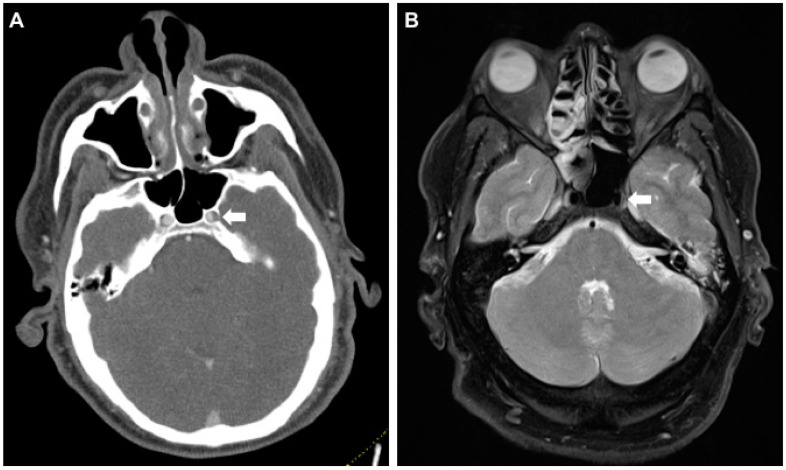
False-positive CT-A raising suspicion for an intramural hematoma of the left internal carotid artery (ICA) in its petrosal segment. (A) Axial CT-A of the skull base shows caliber asymmetry of the left ICA in the foramen carotideum (white arrow). (B) MR (proton-density fat saturated) rules out a wall hematoma. Asymmetry is presumably caused by the carotid plexus of nerves and sympathetics from the superior cervical ganglion (white arrow). MR, magnetic resonance.
